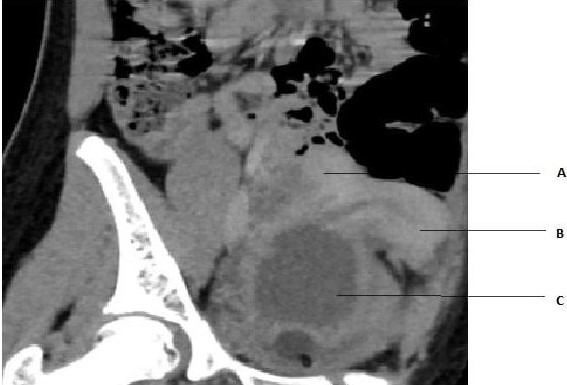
Contrast-enhanced CT scan image showing two separate uteri (A and B) with widely divergent apices, and two separate cervices. Left uterine and cervical cavities are seen in continuity with the hematocolpos (C).
